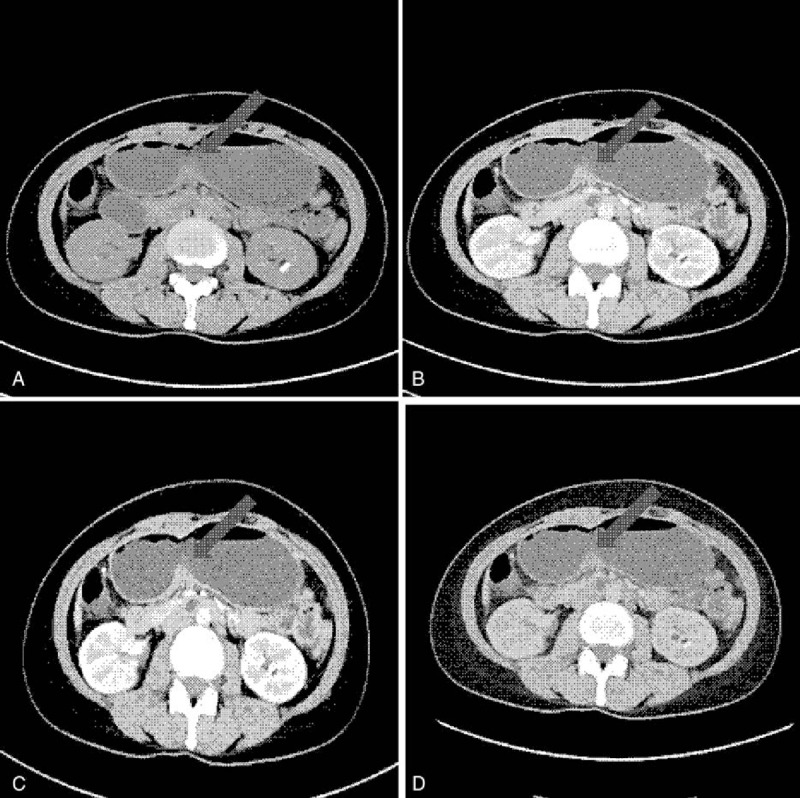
the pictures of intravenous enhanced computed tomography scan of this patient: (A): plain phase; (B): aterial phase; (C): portal vein phase; (D): delayed phase.
